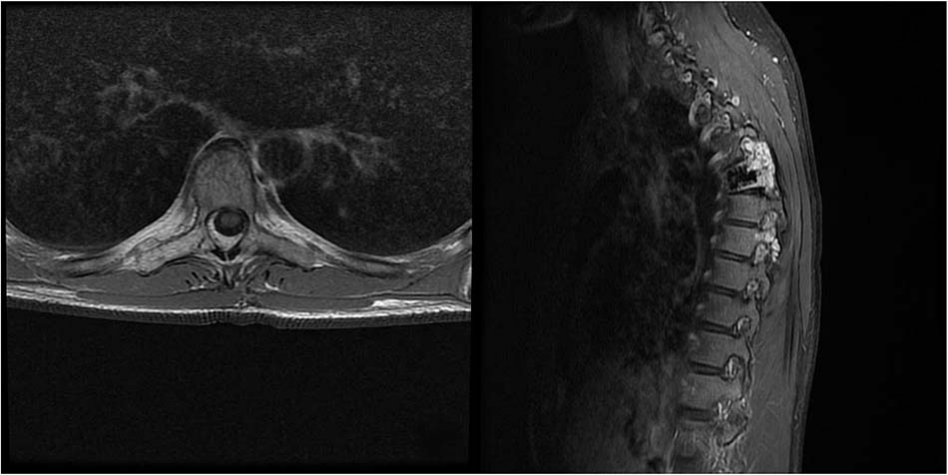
MRI scan of the thoracic spine showing a concomitant haemangioma in the right transverse and articular process of Th8.
